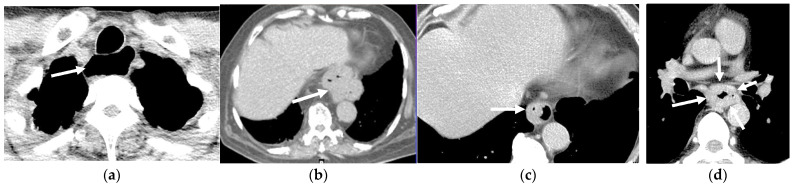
The incidental esophageal findings in low-dose computed tomography (LDCT) scans. (a) The dilated esophagus at the upper third of the thoracic level (arrow) of a 61-year-old male. (b) A conspicuous hiatal hernia in the lower mediastinum (arrow) of a 79-year-old female. (c) Asymmetrical thickening of the distal esophageal wall (arrow) of a 67-year-old male. (d) Circumferential esophageal wall thickening at the middle third of the thoracic level of a 52-year-old male, raising suspicion of esophageal cancer (arrows).
